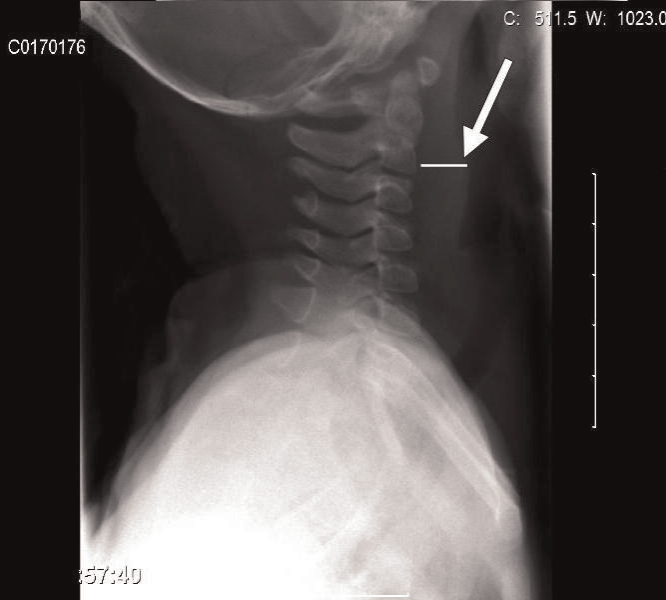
Plain radiograph lateral view of the neck taken at time of presentation showing retropharyngeal abscess.
